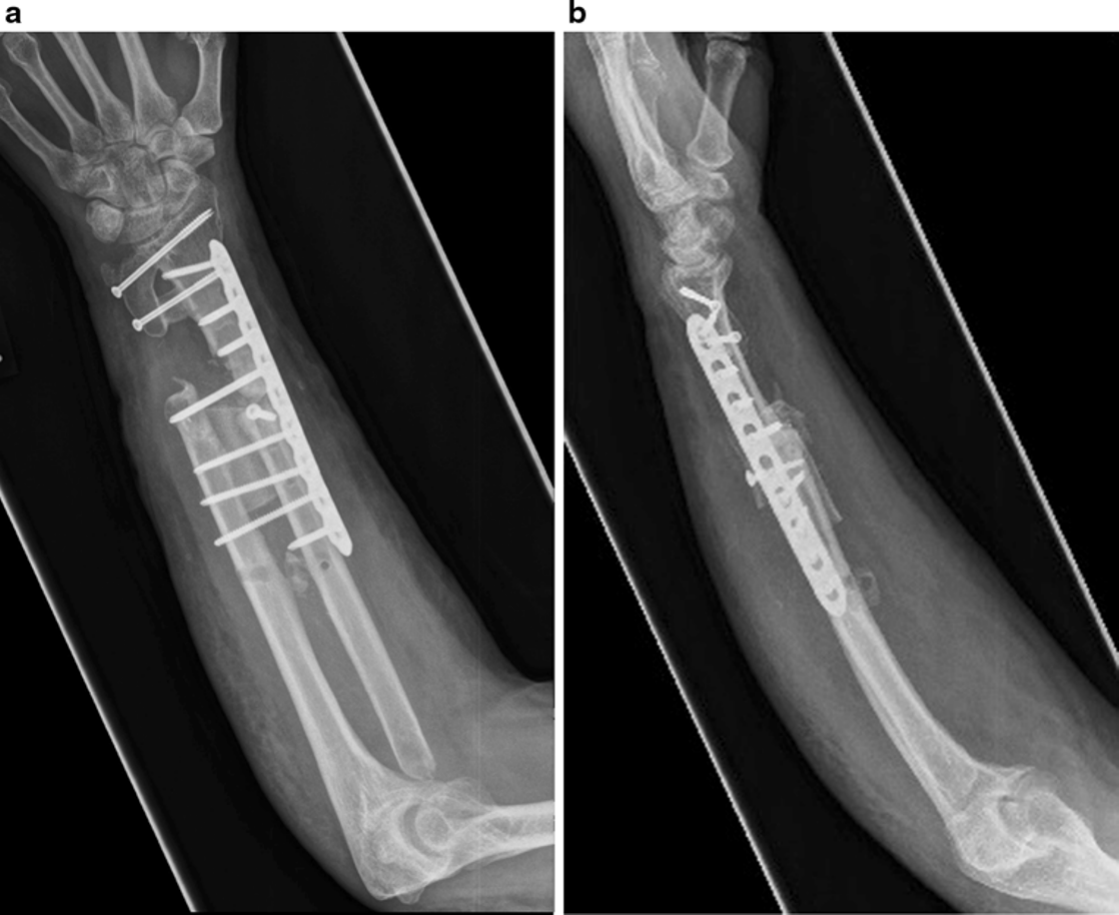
AP and lateral forearm radiographs of the healed one-bone forearm
